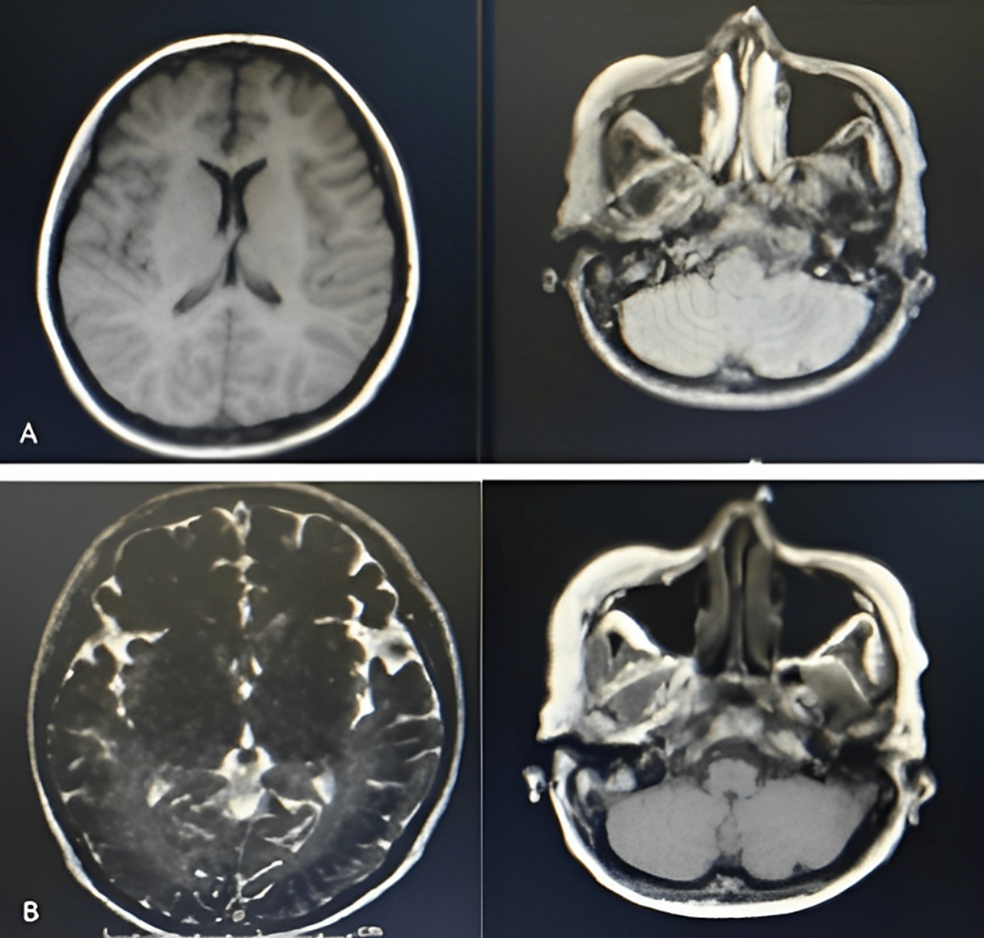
T1 (A) and T2 weighted (B) cranial MRI on the axial view taken during the patient’s first hospital admission showing chronic lacunar infarcts on the right frontal and left cerebellar lobes. MRI: magnetic resonance imaging
